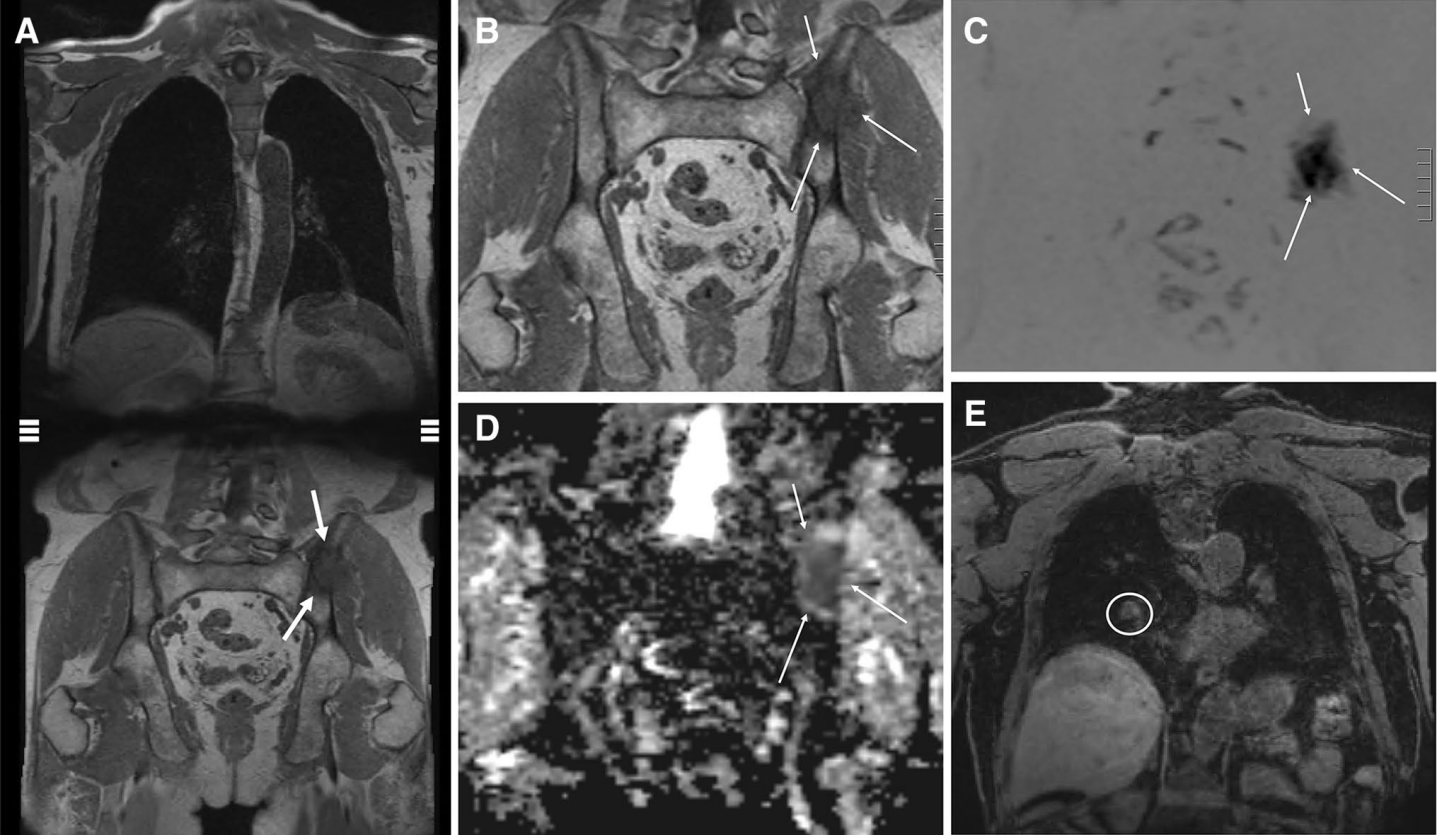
73-year-old man with a Gleason 4 + 3 PCa pT3 with PSA of 18.2 ng/ml. A whole-body MR examination was performed. Coronal whole body T1-weighted image (a) shows a left iliac bone metastasis (b; magnification; arrows). The bone metastasis (arrows) are evident on whole-body DWI images (c, d). e In the right lung, para-hilar, a 15 mm metastasis was observed (circle)
